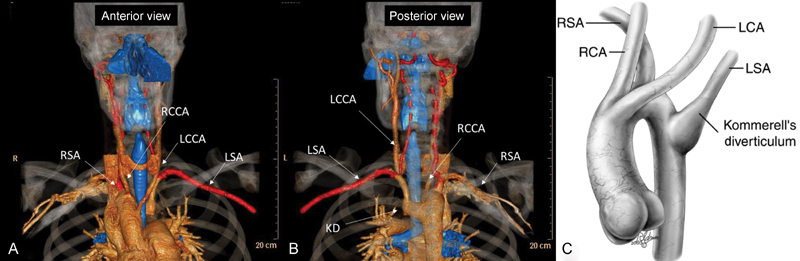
Preoperative computed tomography angiography of the right aortic arch with aberrant origin of LSA. (
